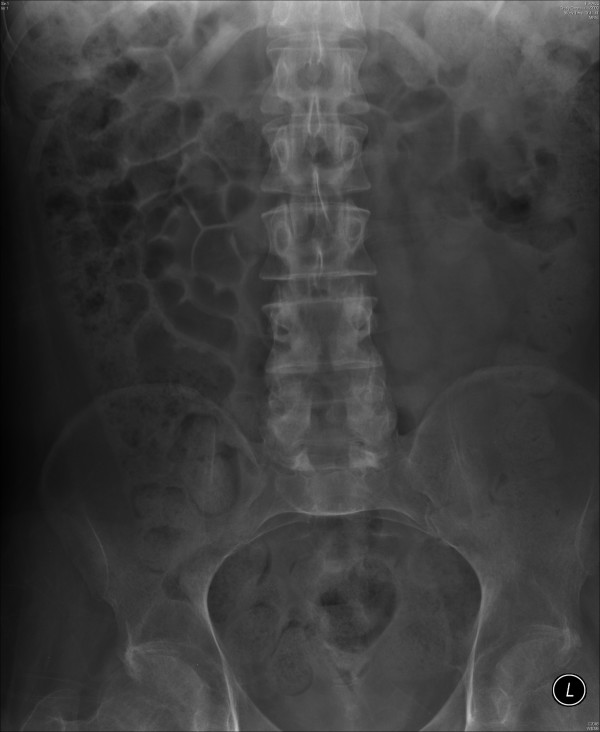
X-ray of abdomen, taken on 11 November 2009, showed faecal loading of the colon.
